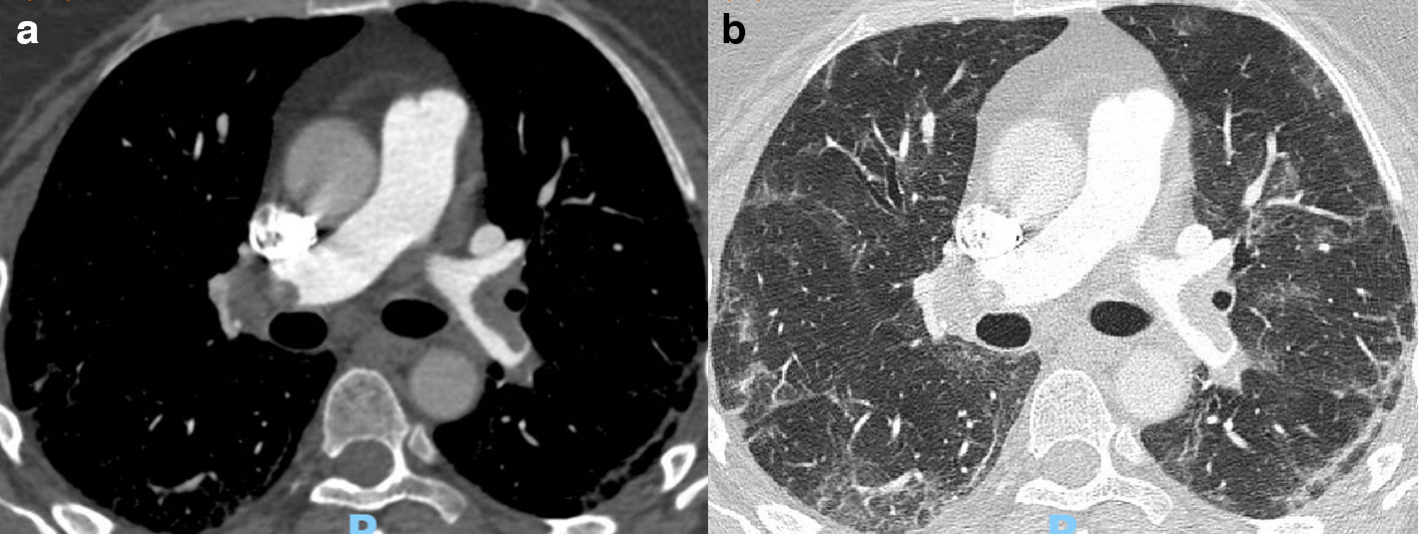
CT pulmonary angiography performed at Emergency Department acceptance in a 62-year-old female with bilateral pulmonary thromboembolism involving lobar, segmental and subsegmental arterial branches. She was subsequently hospitalised, treated with heparin therapy, and discharged after 21 days of hospitalisation, without intensive care need. In (A), the mediastinal setting image shows bilateral pulmonary thromboembolism. In (B), the lung setting shows bilateral ground-glass parenchymal and interstitial involvement, mainly in the posterior segments.
